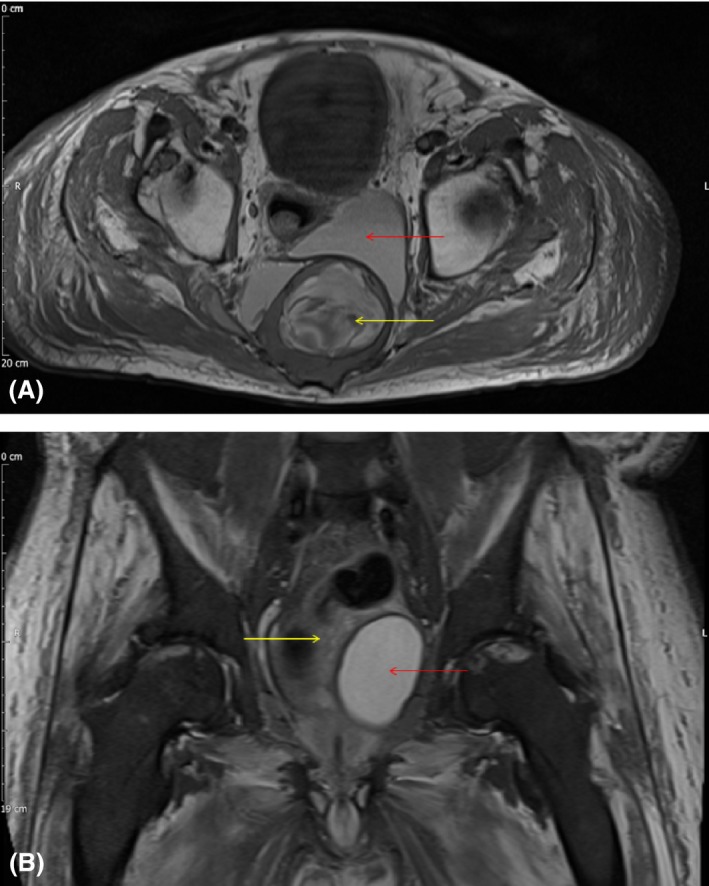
(A and B) T1‐weighted axial magnetic resonance image (A) and T1‐weighted turbo inversion recovery magnitude (TIRM) coronal magnetic resonance image. These demonstrate the large mixed fluid containing (red arrows) and heterogeneous soft tissue (yellow) areas of the presacral pelvic mass consistent with a teratodermoid intimately connected to, and possibly involving the sacrum, with soft tissue extension posterior to the posterior sacral contour.
